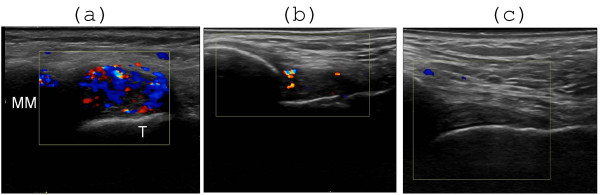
Effect of US-guided steroid injection in the talo-crural joint as revealed by color Doppler . (A) Before steroid injection there is synovial hyperemia anterior to the medial malleolus (MM). T = talus. (B) One week after steroid injection there is partial regression of hyperemia. (C) Normalization with complete regression of hyperemia 4 weeks after steroid injection.
